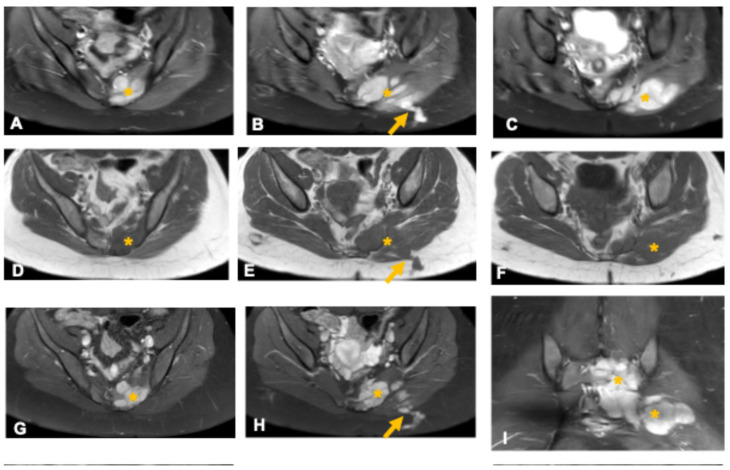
Sequential axial and coronal MR images (A–I) demonstrate an expansile lesion (asterisk) involving the lower left sacrum. The lesion shows predominantly high signal intensity on T2-weighted fat-suppressed images (A–C), low signal intensity on T1-weighted sequences (D–F), and diffuse heterogeneous enhancement on post-contrast fat-suppressed images (G–I). It has lobulated margins and extends extraosseously into the gluteal muscles and subcutaneous tissue, following the course of vascular structures, including perforating vessels (arrows). The elongated configuration and perivascular extension are suggestive imaging features of PEComa, although findings remain nonspecific.
